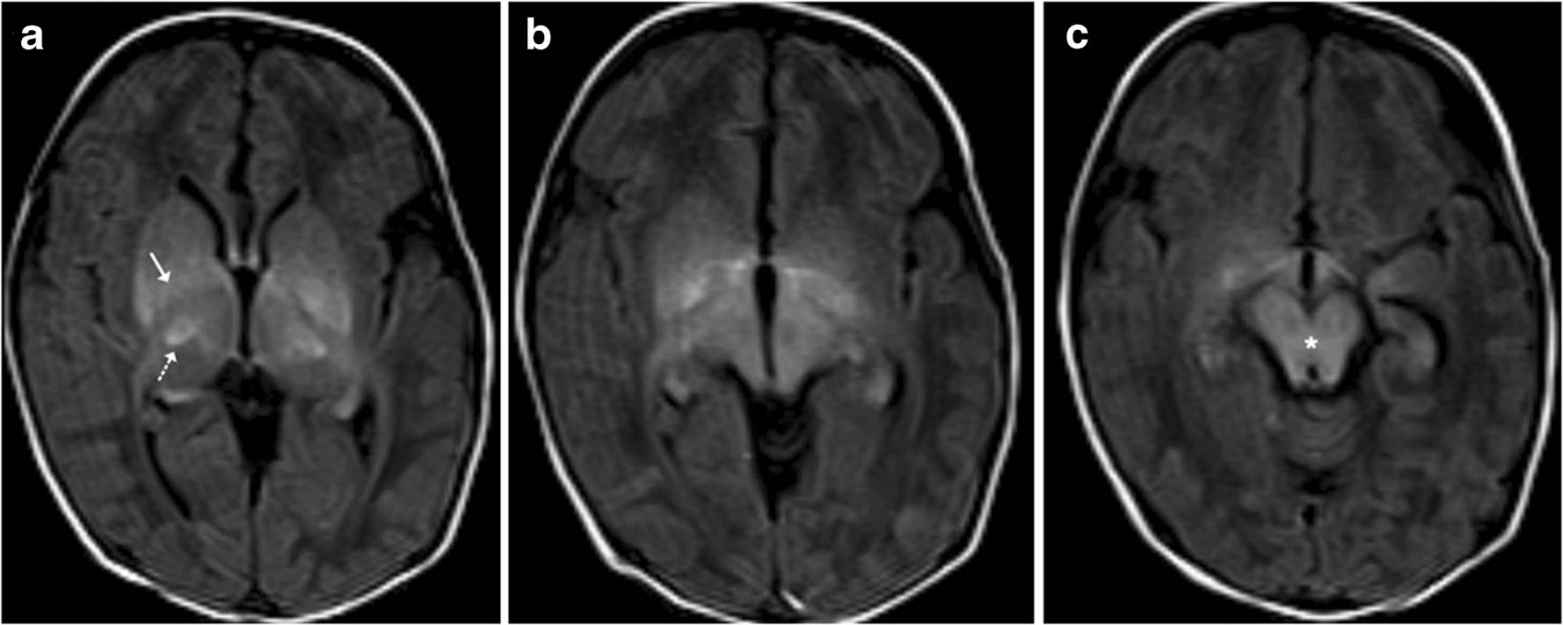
Neonate with a history of delayed cry: a T1-weighted image shows symmetric increased signal intensity involving the putamina (*arrow*) and ventrolateral thalami (*dotted arrow*). b–c Similar high signal intensity is also noted involving the midbrain (*asterisk*)
